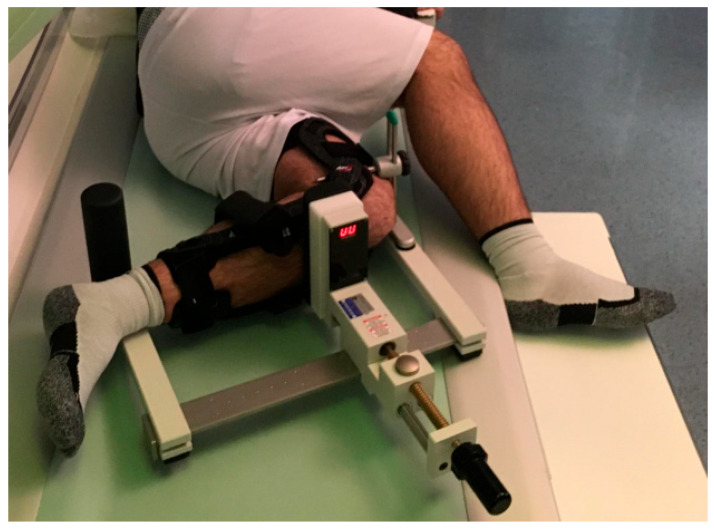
Patient position during stress radiography; with the knee in 90° flexion, a posterior stress radiograph was performed with use of the Telos device.
